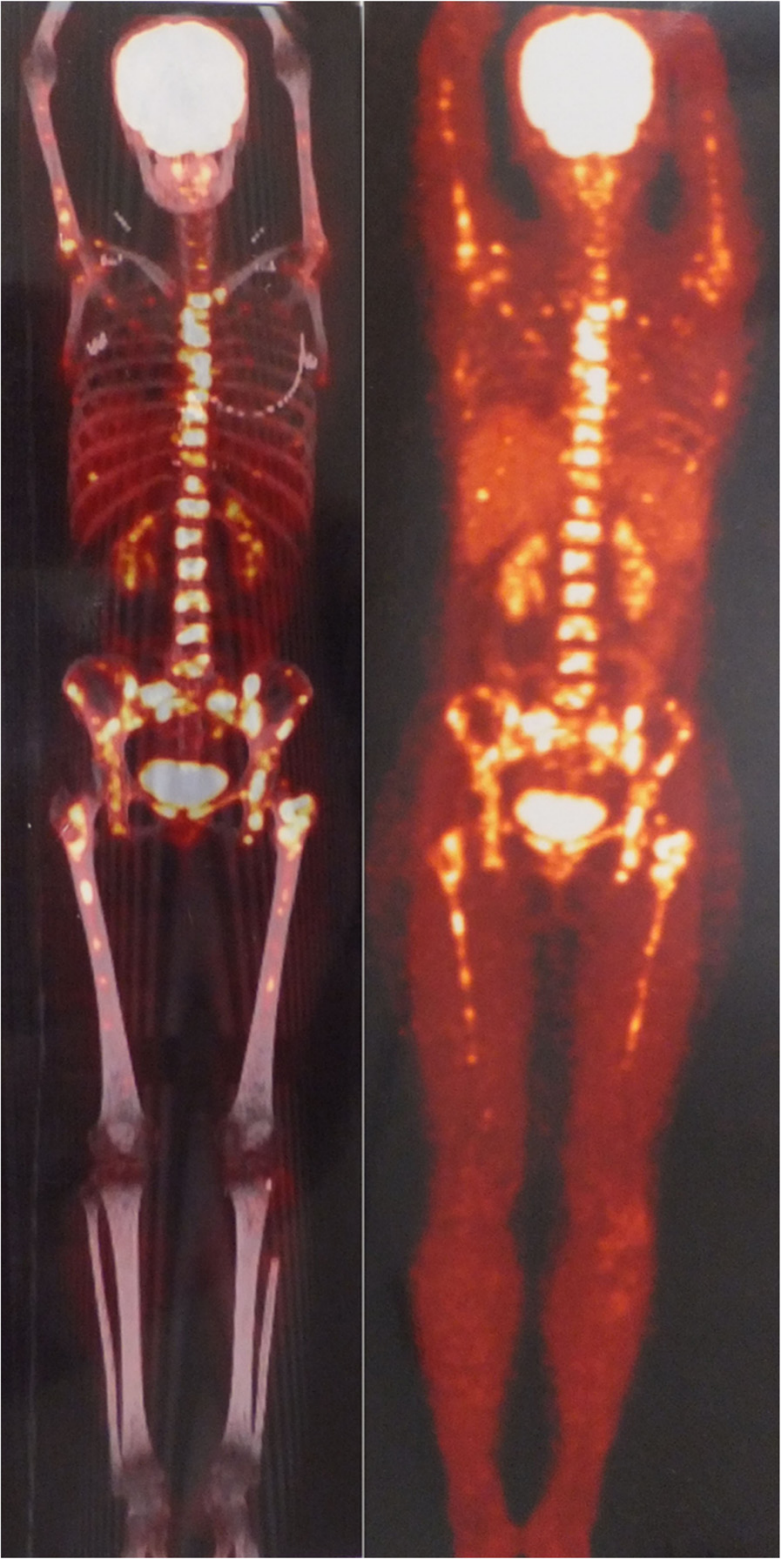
PET/CT of the patient. F-18 FDG PET/CT shows multiple bone metastases especially and symmetrically in the axial skeleton and proximal extremities including bilateral femurs and humeri, with a maximum SUV of 10.8, but with no signs of local recurrence and no focal F-18 FDG uptake in the brain, head, neck, chest, and abdomen except a small nodule in the liver which was suspected as a calcification.
